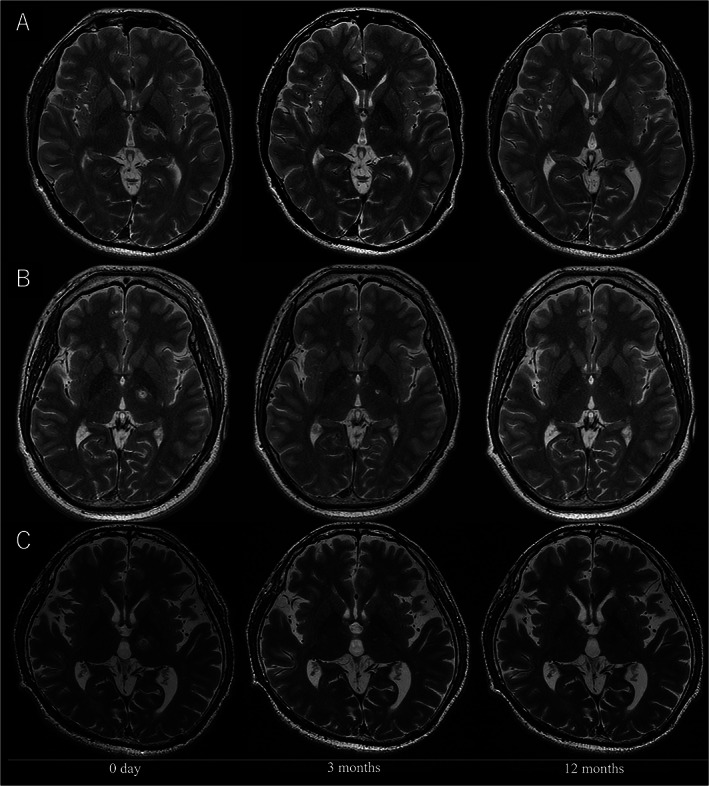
Representative magnetic resonance images obtained before and after treatment. The lesion unexpectedly encroached on the posterior limb of the internal capsule (case 4: A, case 7: B). Case 9 (C) showing the precise lesion on the intended target.
